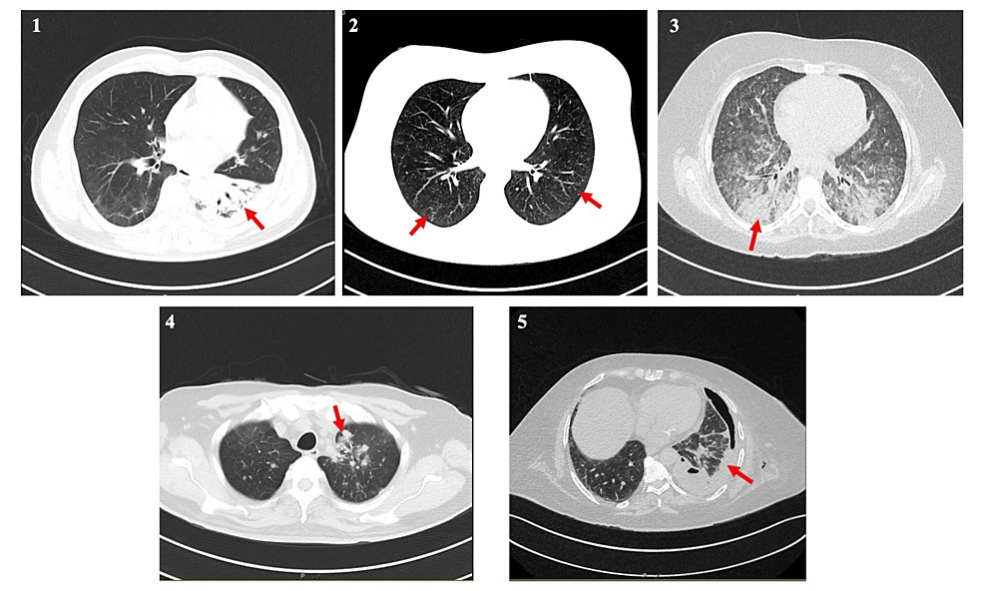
High-resolution computed tomography scan of the patients after developing post-COVID tuberculosis Case 1: Dense consolidation of left lower lobe. Case 2: Diffuse miliary nodules. Case 3: Patchy infiltrates present in both lower lobes. Case 4: Nodular infiltrates present in the left upper lobe. Case 5: Left lower cavitary consolidation complicated with left hydropneumothorax.
